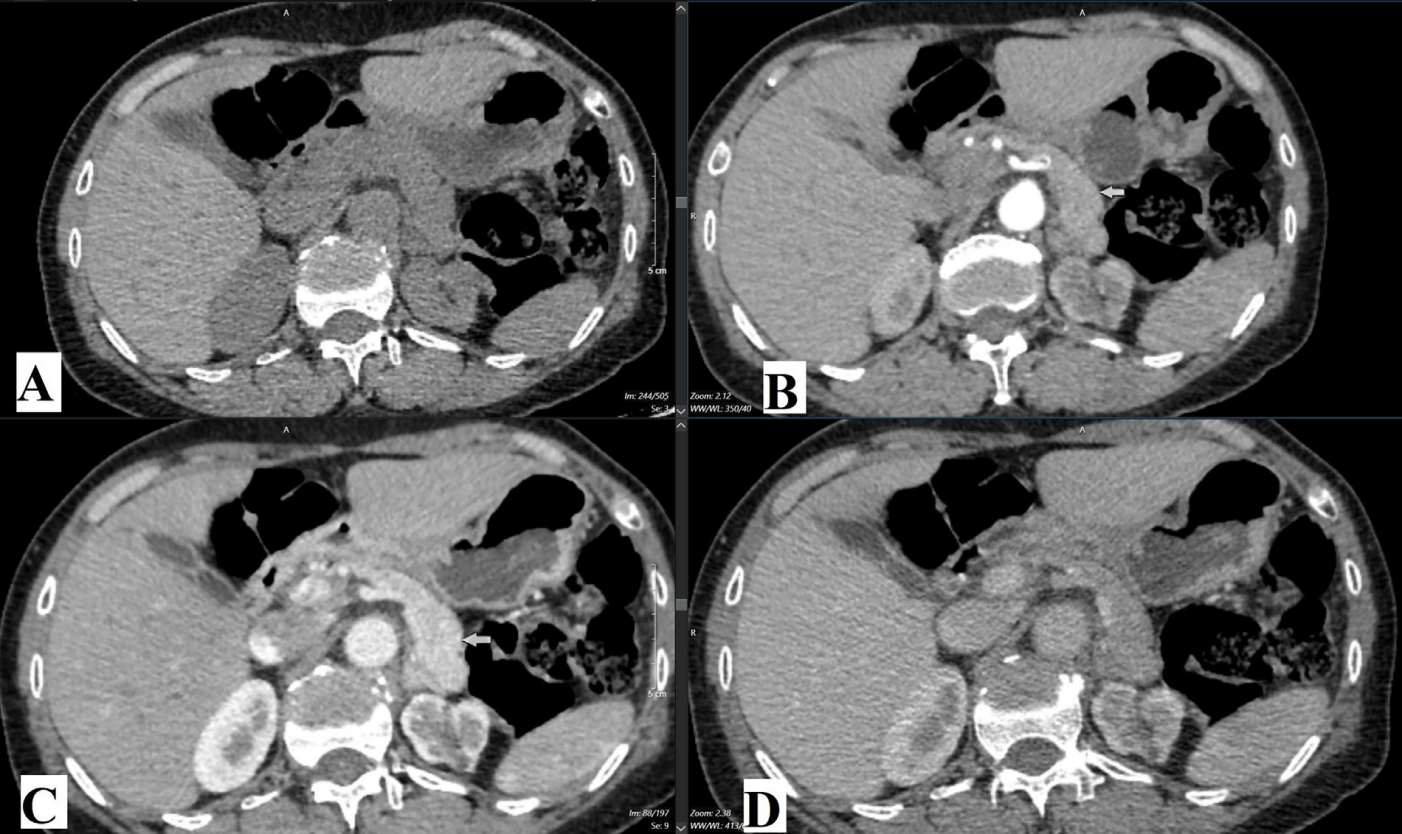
Contrast-enhanced computed tomography (CT) images at different phases: (A) noncontrast phase, (B) arterial phase, (C) portal venous phase, and (D) delayed phase. White arrow in (B) arterial phase, (C) venous phaseindicates the approximate anatomical region of interest (between body and tail of the pancreatic), with no evident contrast enhancement suggestive of a lesion.
